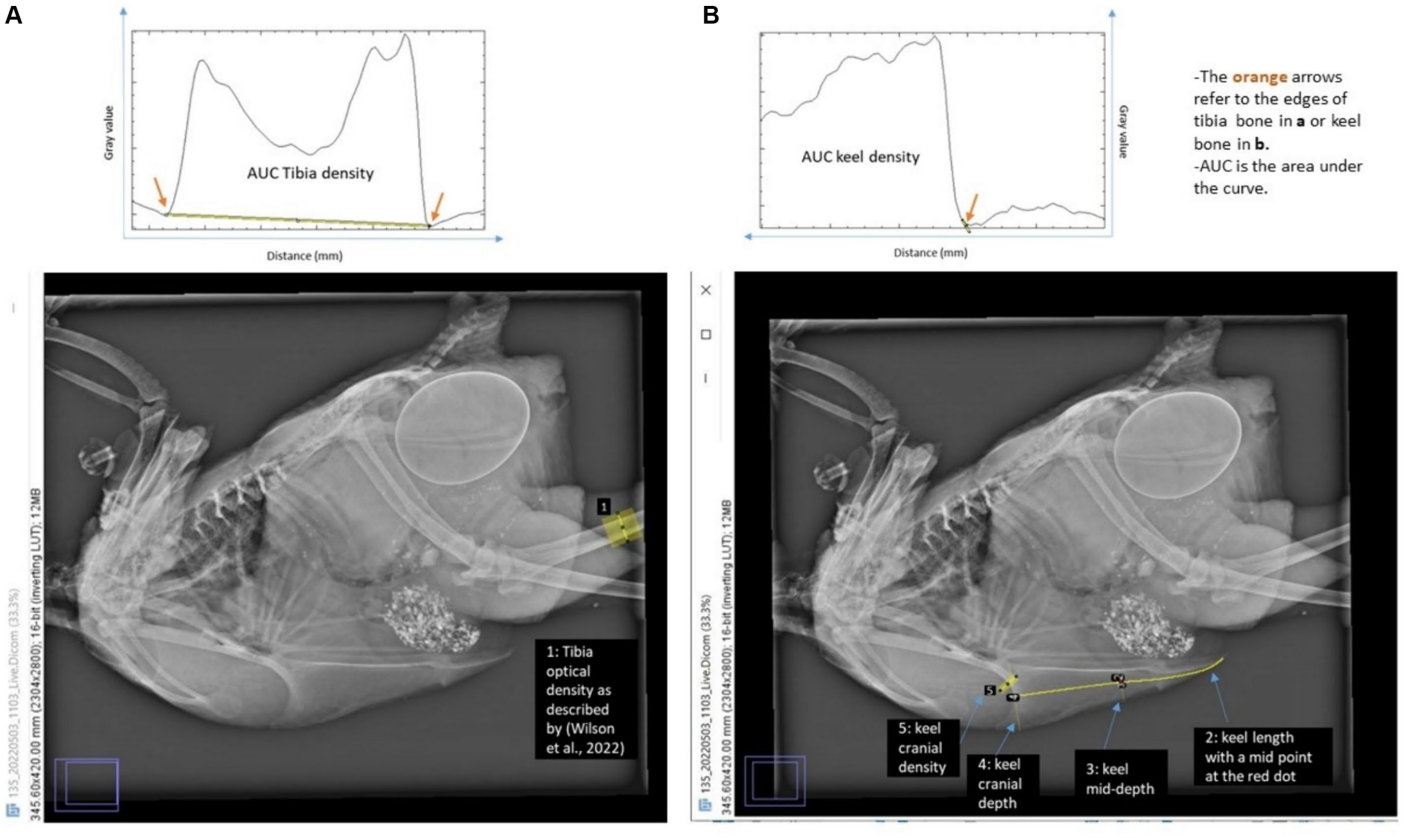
Measurement locations on radiography using the ImageJ program and estimated density of (A) tibiotarsal and (B) keel bone. Orientations: for the body and keel bone (cranial to the left, caudal to the right, dorsal at the top of image, ventral at the bottom of image) and for tibiotarsal bone (cranial to the bottom of image, caudal to the top of image, proximal at the left of image, distal at the right of image).
